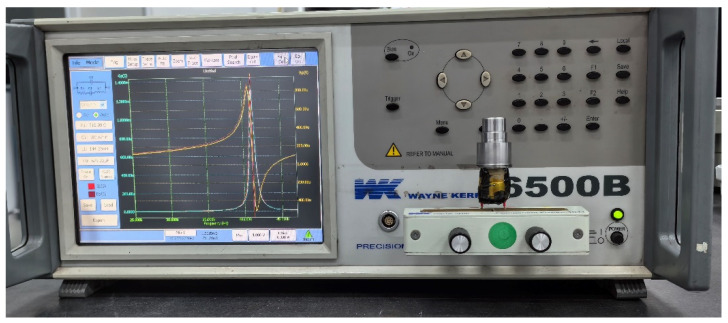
Impedance testing setup for the ultrasonic transducer.
